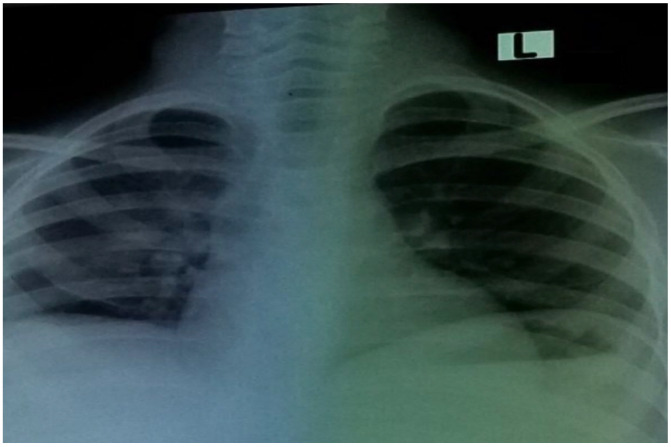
Chest X-ray of patient showing resolution of right pleural effusion on hospital admission day 7.
